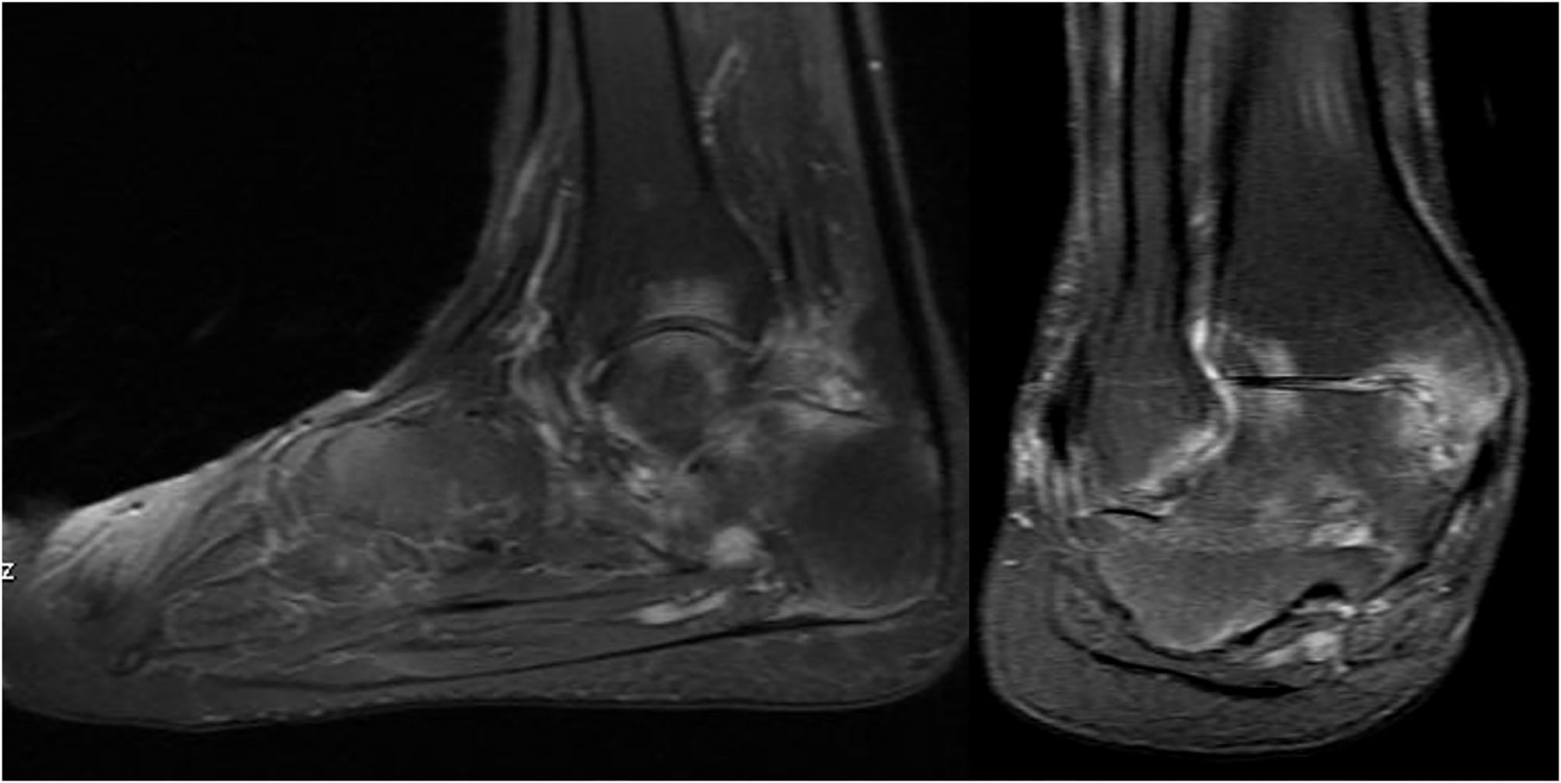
T-2 weighted-fat suppressed MR imaging showing edema in various tarsal bones, joint spaces and soft tissues in a 16 year old boy with chronic ankylosing tarsitis [Modified from Burgos-Vargas (46)].
